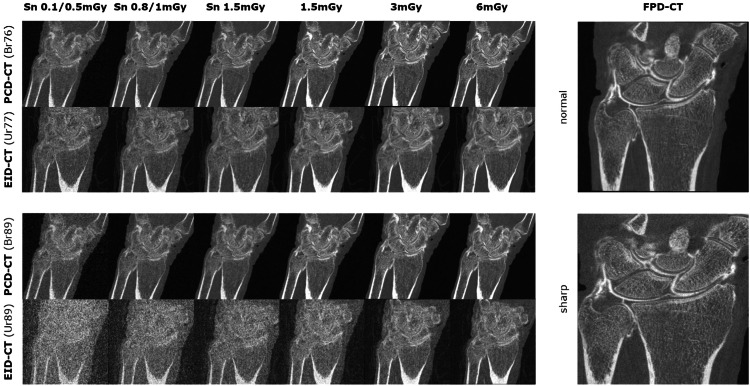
Exemplary images of all dose parameters and reconstruction kernels in the PCD-CT, EID-CT, and FPD-CT. CT, Computed tomography; EID-CT, Energy-integrating detector CT; FPD-CT, Flat-panel detector CT; PCD-CT, Photon-counting detector CT
